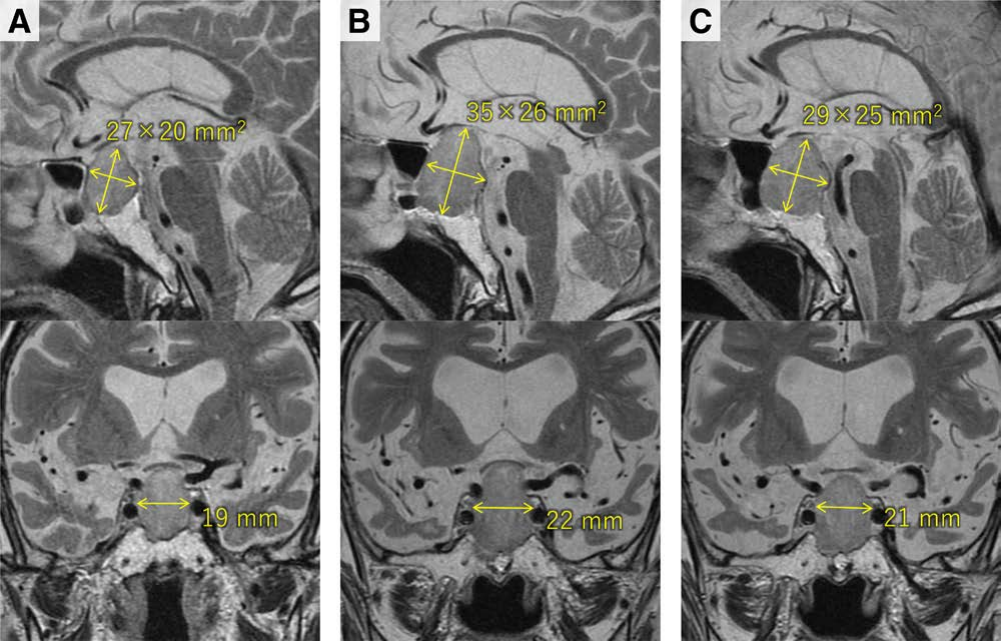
The findings of magnetic resonance imaging of the pituitary tumor. (A) The size of the pituitary tumor measured 27 × 20 × 19 mm^3^ when the last follow-up MRI was performed about 9 years before the first admission to our hospital. (B) The tumor enlarged to 35 × 26 × 22 mm^3^ at the time of the first admission. (C) The pituitary tumor shrank slightly (29 × 25 × 21 mm^3^) after 1 year of cabergoline administration at a weekly dose of 0.25 mg. MRI = magnetic resonance imaging.
